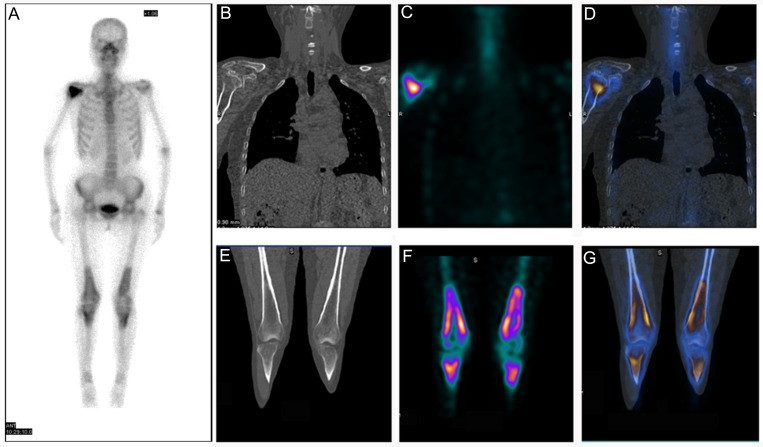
Patient 3: (A) ${}^{99m}$Tc-MDP bone scan demonstrated symmetric uptake of radiotracer around both knee joints as well as abnormal tracer uptake in the right humeral head. (B–D) Coronal CT image revealed “Double line sign” accompanied by increased tracer uptake under articular surface of the corresponding humeral head. (E–G) CT, SPECT and fusion image of the patient’s bilateral tibiae showed abnormal tracer uptake with seemingly normal CT appearance.
